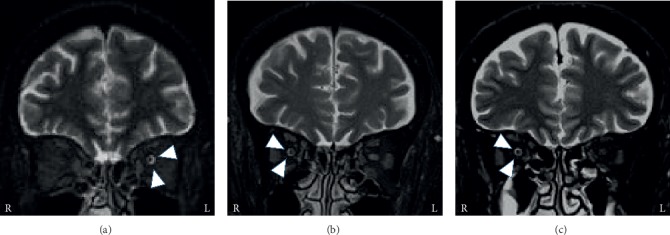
Brain MRI of cases 2–4. In case 2 (a), the high-intensity ring appearance (arrowheads) around the left optic nerve is found on STIR MRI. In cases 3 (b) and 4 (c), the high-intensity ring appearance (arrowheads) around the right optic nerve is found on fat-suppressed T2-weighted MRI.
